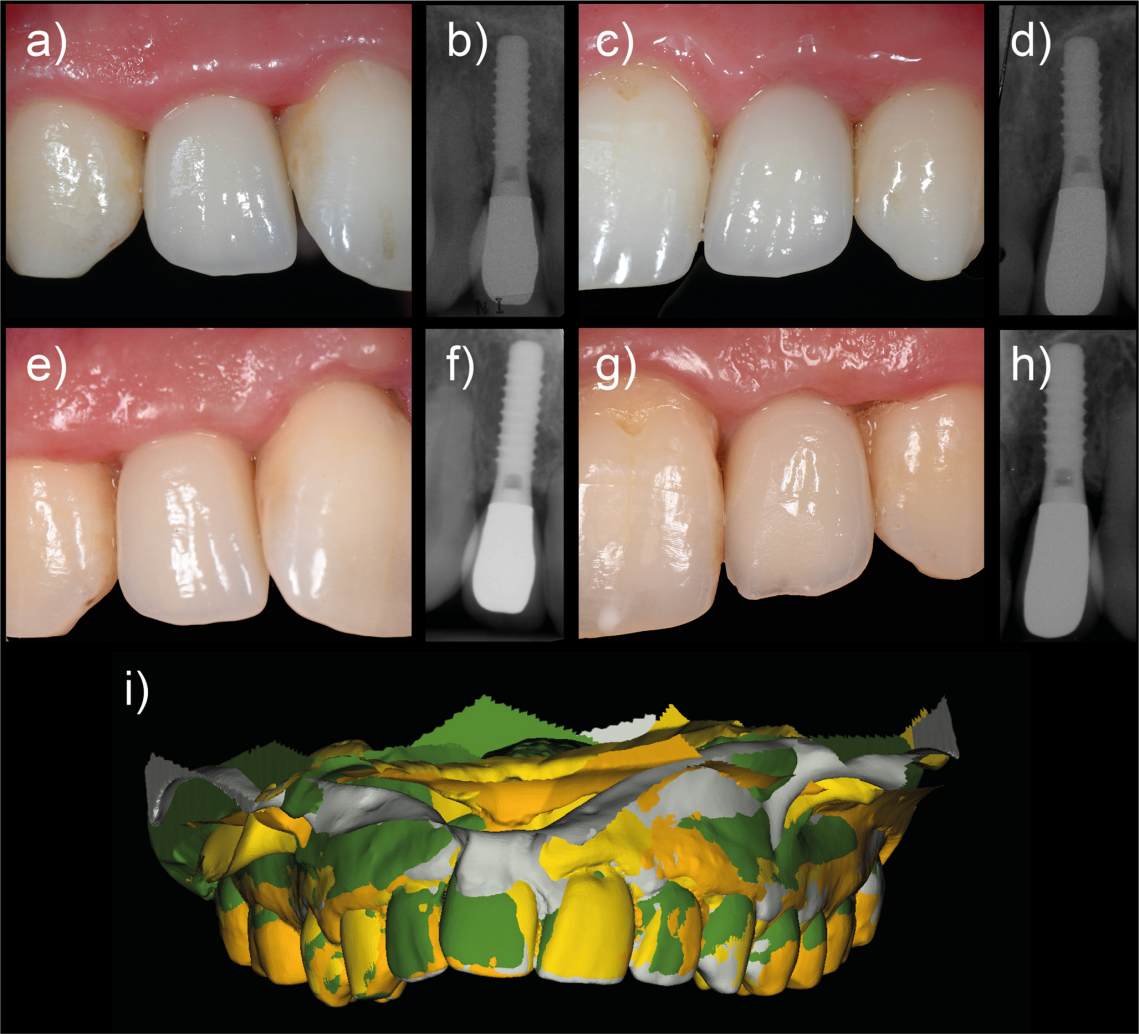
Clinical images and X-rays at different follow-up appointments: (a) right lateral incisor at 1-year follow-up, (b) X-ray at 1-year follow-up, (c) left lateral incisor at 1-year follow-up, (d) X-ray at 1-year follow-up, (e) right lateral incisor at 12-year follow-up, (f) X-ray at 12-year follow-up, (g) left lateral incisor at 12-year follow-up, (h) X-ray at 12-year follow-up, and (i) superimposition of the models
